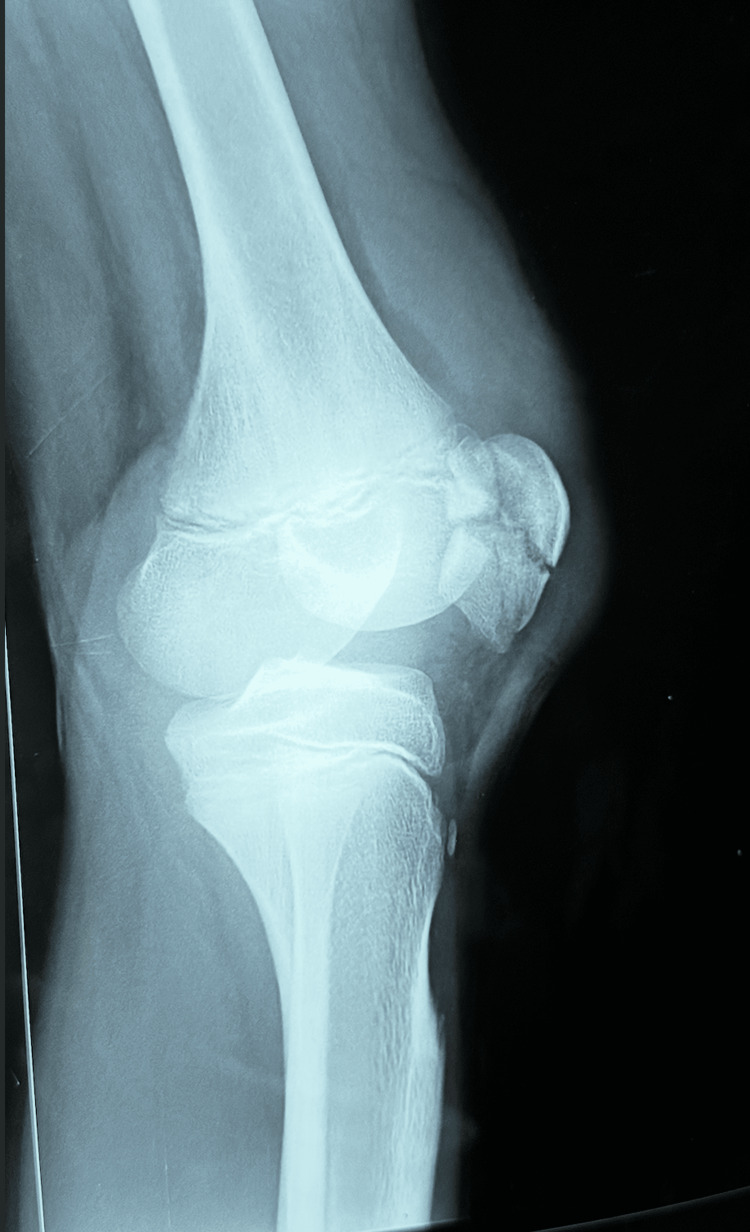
Preoperative X-ray showing a comminuted patellar fracture
